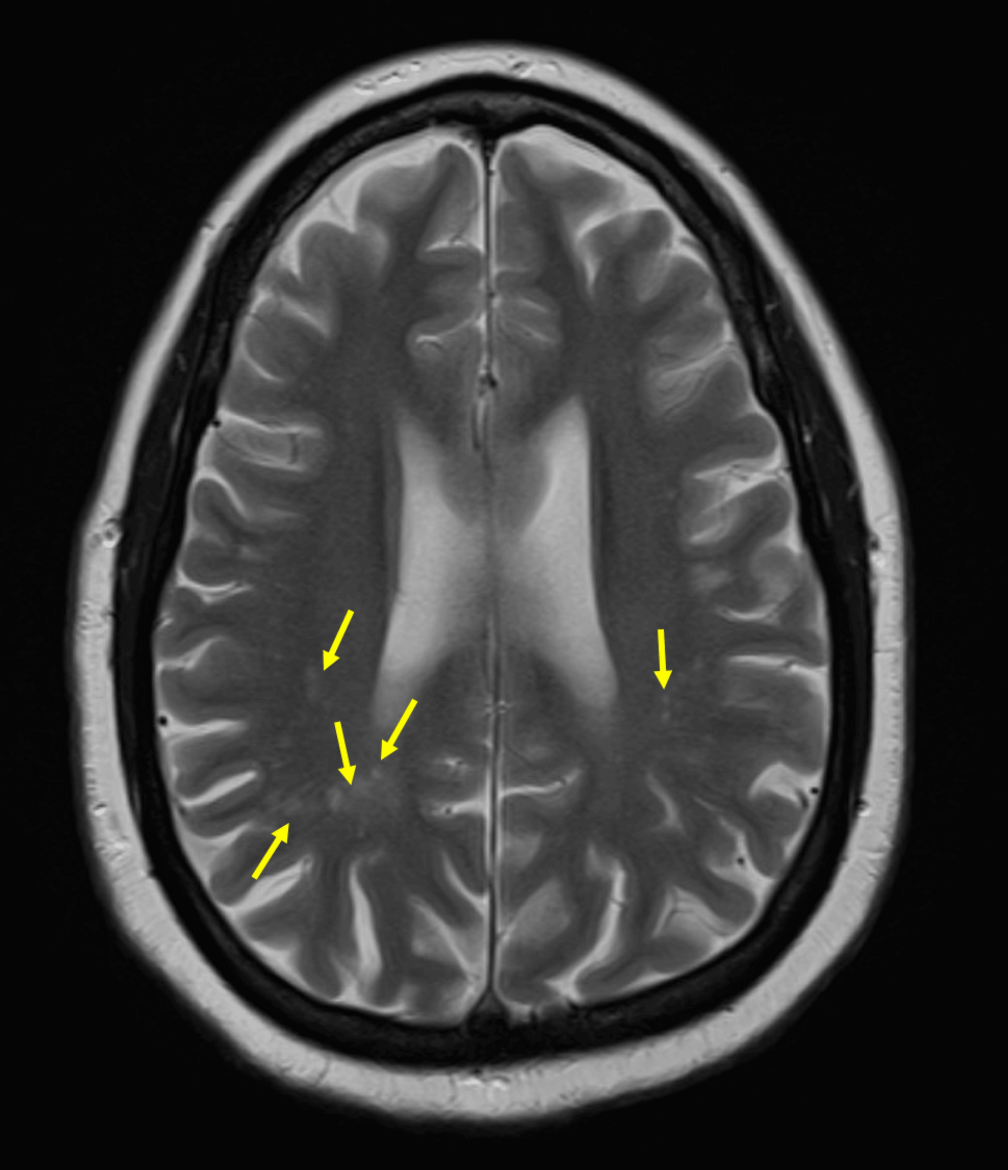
Multiple subcortical white matter lesions. Note the multiple subcortical white matter hyperintense lesions (arrowheads) on the T2-weighted image of brain MRI without contrast.
